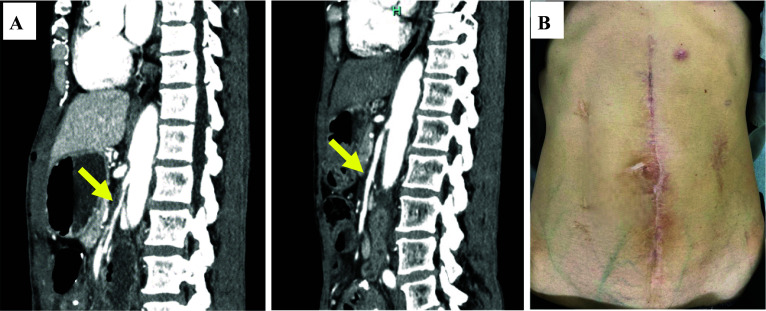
Postoperative course is depicted. A Computed tomography (CT) indicating narrowing of the superior mesenteric artery (SMA) with dissociation (left). At 7 months postoperatively, CT reveals that the narrowing of the SMA has resolved (right). B Surgical scar is depicted
